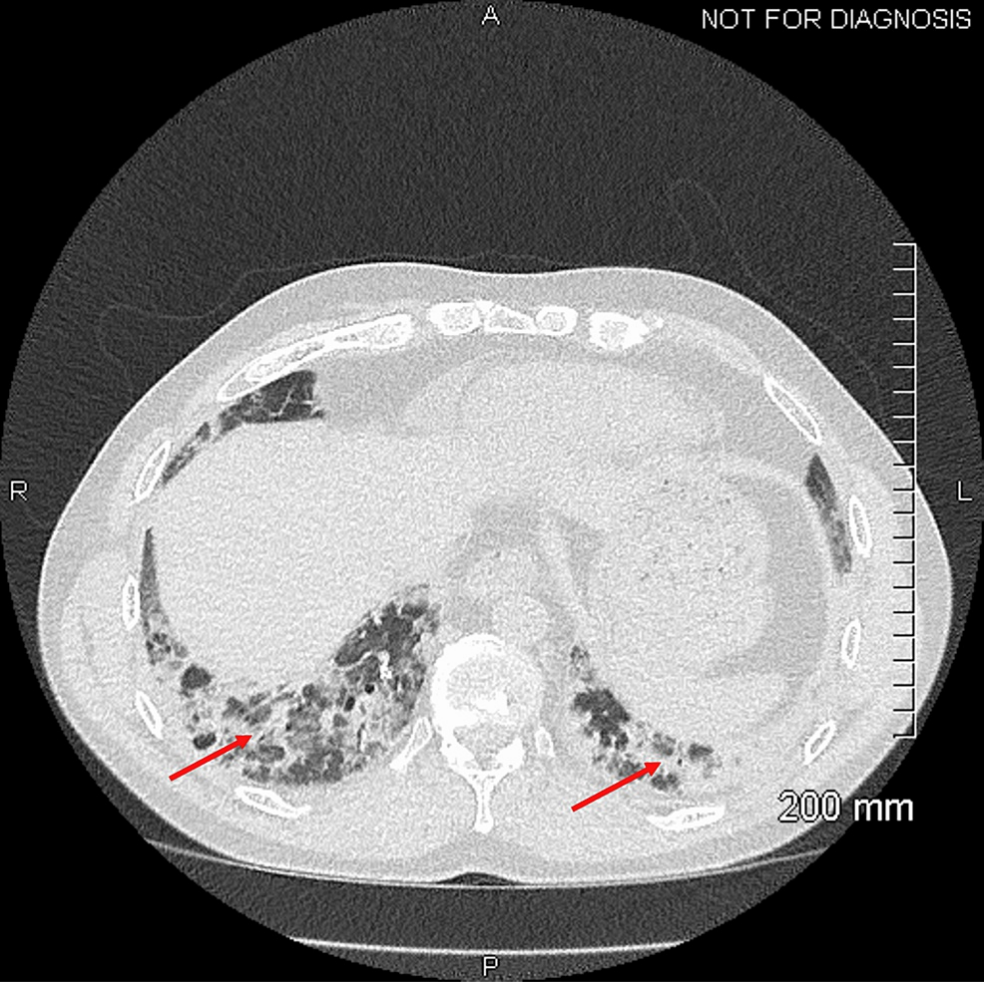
Non-contrast CT chest Axial view with bibasilar ground-glass opacities as indicated by arrows CT: computed tomography
